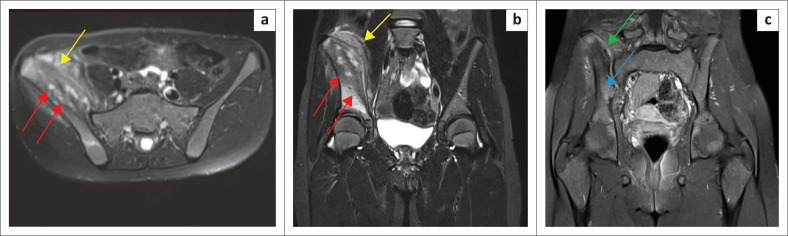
Magnetic resonance imaging of the pelvis performed without and with gadolinium: (a) Axial T2 fat-saturated image of the pelvis showing heterogeneous marrow signal within the right iliac wing with marrow expansion (red arrows). Diffuse layered periosteal reaction was noted with increased T2 signal in the adjacent part of the iliacus and gluteal muscles (yellow arrow). (b) Coronal short tau inversion recovery (STIR) image of the pelvis showing layers of periosteal reaction (yellow arrow). Increased marrow signal could be seen extending close to the acetabular roof but without intra-articular extension (red arrows). (c) Coronal fat-saturated image gadolinium enhanced T1 weighted image indicated mild sacroiliitis with post contrast enhancement (green arrow) and heterogeneous marrow enhancement (blue arrow). In addition, there was enhancement of the adjacent muscles.
